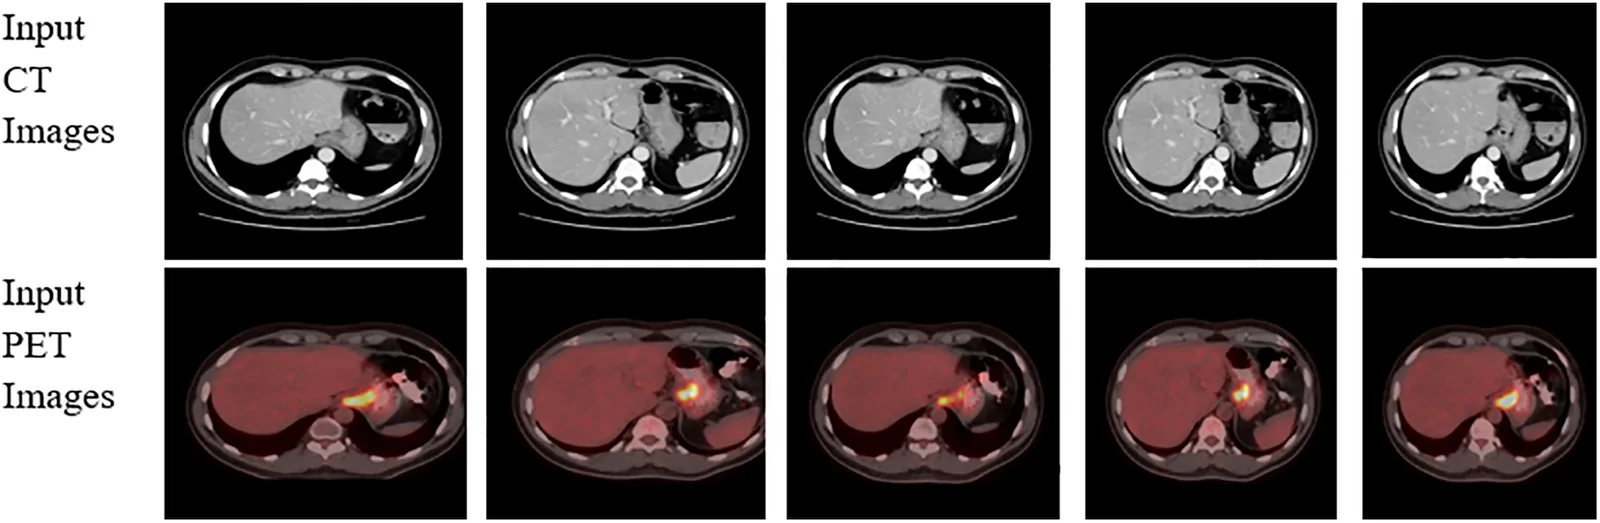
Sample gist CT-PET images from data set.
